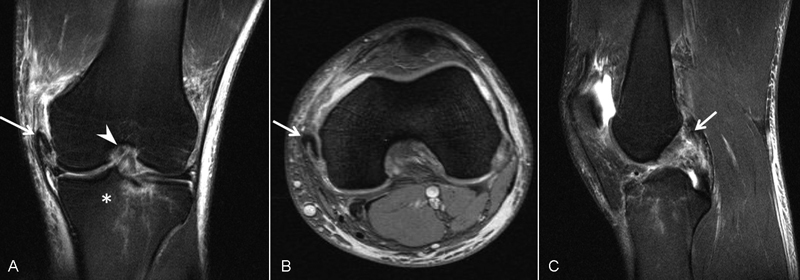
T2-weighted magnetic resonance imaging of the injured knee. (
